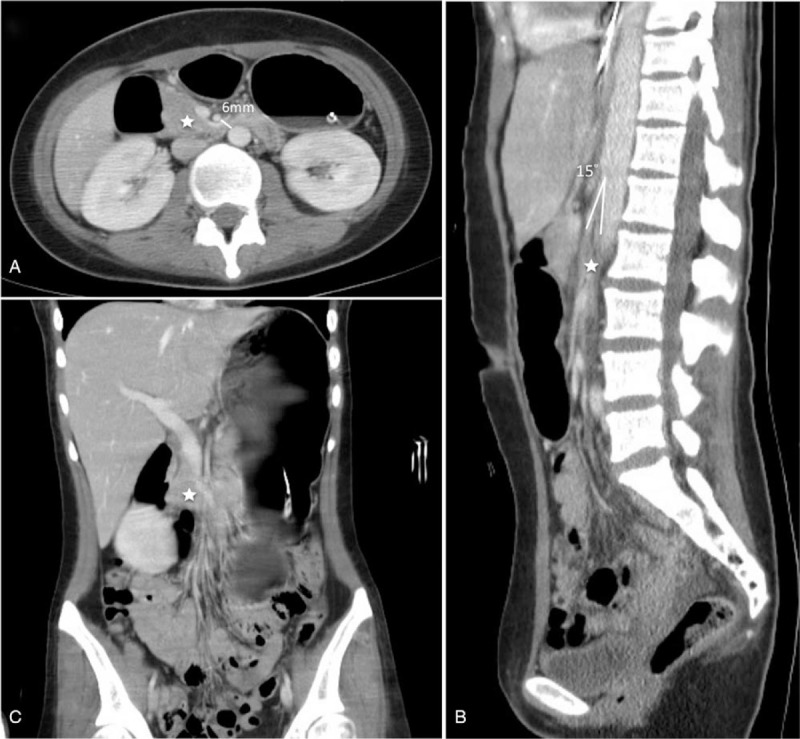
A brain MRI showed bilateral extensive signal hyperintensity in T2-weighted imaging, indicating a previous insult of cerebellar hemispheric infarction at SCA and PICA territories bilaterally. PICA = posterior inferior cerebellar artery, SCA = superior cerebellar artery, MRI = magnetic resonance imaging.
